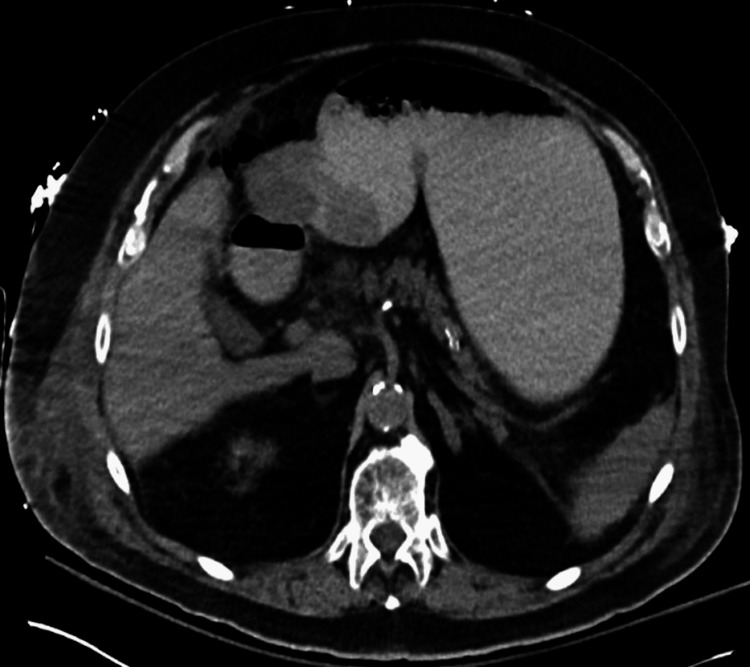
Abdominal CT-Axial. Axial without contrast, showing free air and fluid surrounding the transverse colon, due to a possible perforated sigmoid diverticulitis vs post-surgical air and fluid. CT: Computed tomography
